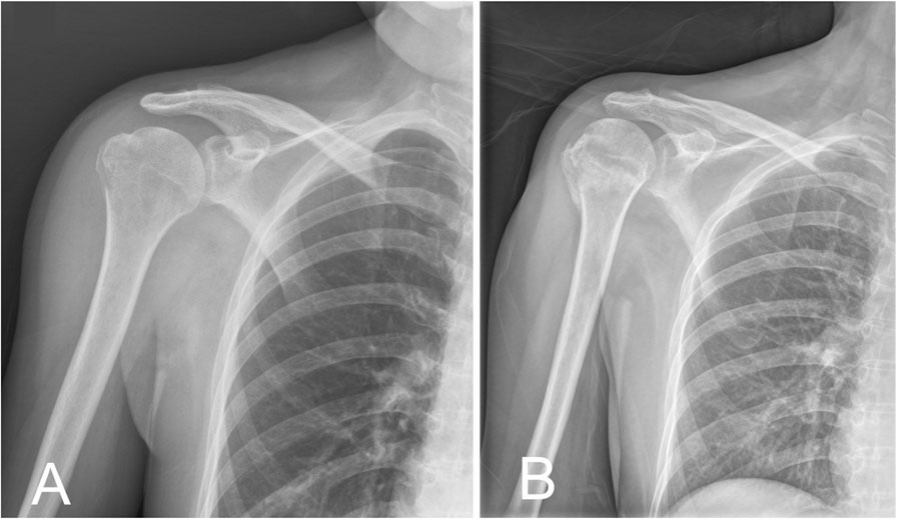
Radiographic findings of occult surgical neck fracture in a patient. a Isolated GT fracture was diagnosed from the radiograph on day 1. b On day 13, radiopacity at the surgical neck implied periosteal new bone formation, which indicated the existence of the occult surgical neck fracture
